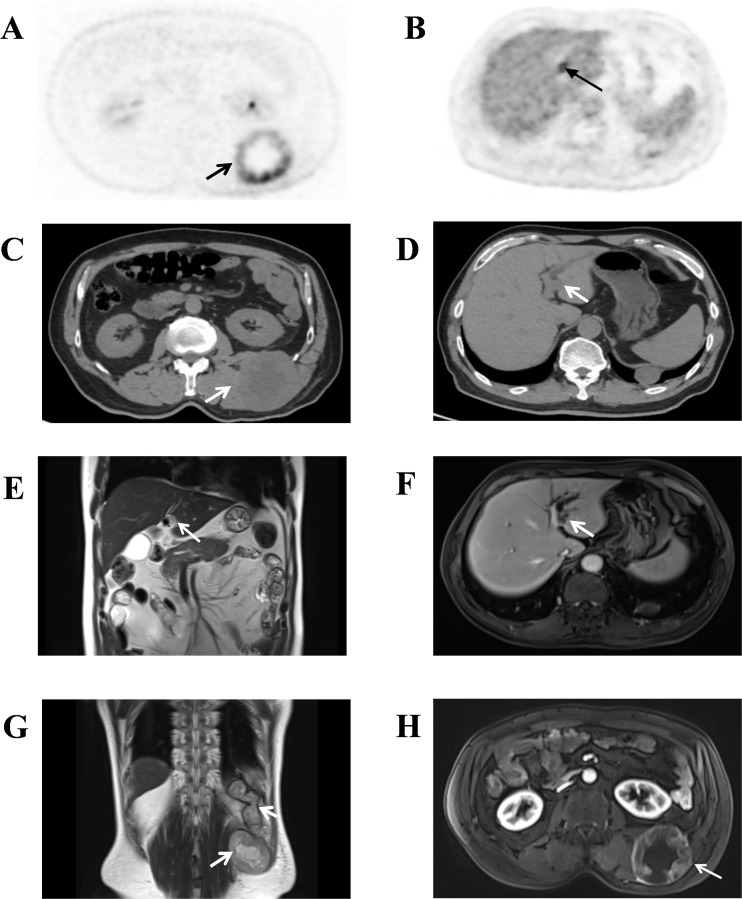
Pertinent imaging studies of the patient. (A-D) PET-CT demonstrated hypermetabolic masses in the left dorsal subcutaneous tissue(SUVmax11.0) (A, C) and the left hepatic lobe(SUVmax4.1) (B, D-H) Coronal T2-weighted imaging (T2WI) and axial arterial phase contrast-enhanced T1-weighted imaging (T1WI) magnetic resonance imaging (MRI) of the liver (E, F) and retroperitoneal masses (G, H). The longest dimension of the hepatic tumor was approximately 1.5 cm, it exhibits a slightly prolonged T2WI signal and shows relative hypointense enhancement compared to normal liver parenchyma. The vertical dimension of the sarcoma was approximately 13.2 cm, with a maximum cross-sectional size of approximately 7.0 × 6.4 cm; T2WI shows mixed signal intensity. On T1-weighted contrast-enhanced scans, there is a central area of non-enhancing necrosis, and the peripheral solid component demonstrates heterogeneous enhancement.
